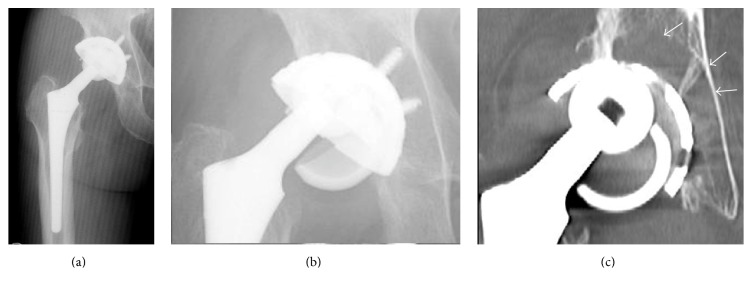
A postoperative anteroposterior radiographic image showing primary THA with alumina-on-alumina bearings on the right side for the treatment of secondary osteoarthritis due to developmental dysplasia of the hip (a). A plain radiograph and a computed tomography (CT) image, obtained 10 years after primary THA, showing the liner's dissociation from the metal shell along with retroacetabular osteolysis (white arrows) (b and c).
